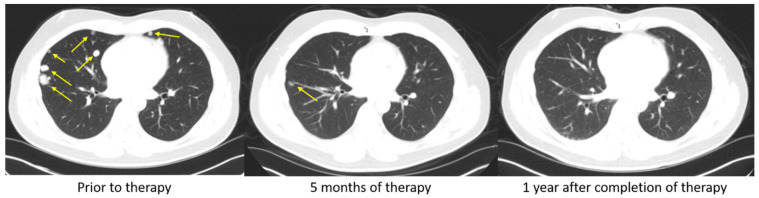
Complete response to PD-1 immune checkpoint inhibitors. A 48-year-old man with HBV cirrhosis developed a liver mass that was biopsied and found to be HCC. He underwent a laparoscopic partial right hepatectomy. Pathology revealed a poorly differentiated HCC, 3.3 cm in size, and margins were negative. On surveillance, he was found to have developed a rising AFP and to have bilateral lung nodules (Figure), but there was no evidence of recurrent or metastatic disease in the abdomen or pelvis. Biopsy of one of the pulmonary nodules confirmed metastatic disease and he was started on sorafenib. However, his disease progressed. He had no FGFR alteration, so he was started on nivolumab. After only 5 months of therapy, an objective response was seen on CT scan (middle). He completed a full year of therapy, and he remains without evidence of disease one year after completion of therapy.
